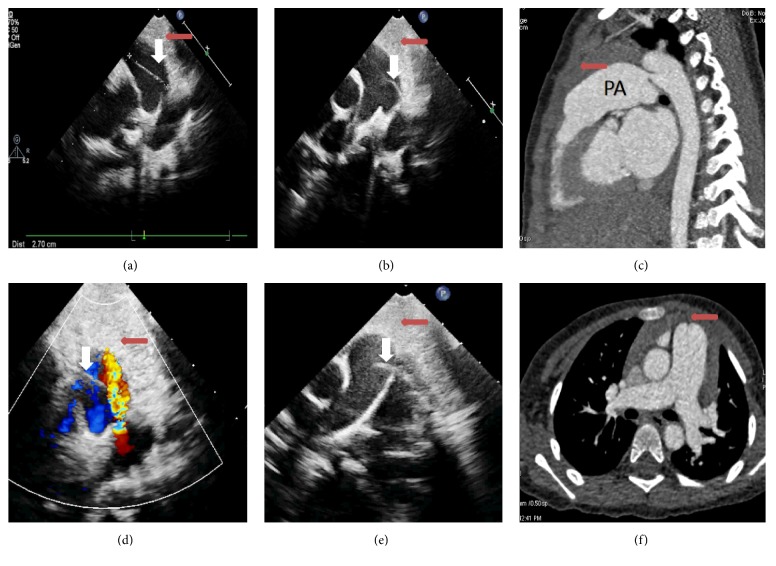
(a, b) Left high parasternal view. (c, f) Computed tomographic pulmonary angiography shows a dilated pulmonary artery (PA) but no artifacts. (d) Color Doppler imaging with patent ductus arteriosus flow. (e) Near-suprasternal aortic short-axis view. A linear artifact is clearly seen ((a, b, d, e): white arrowheads). The thymus gland is seen ((a, b, e, f): red arrowheads).
